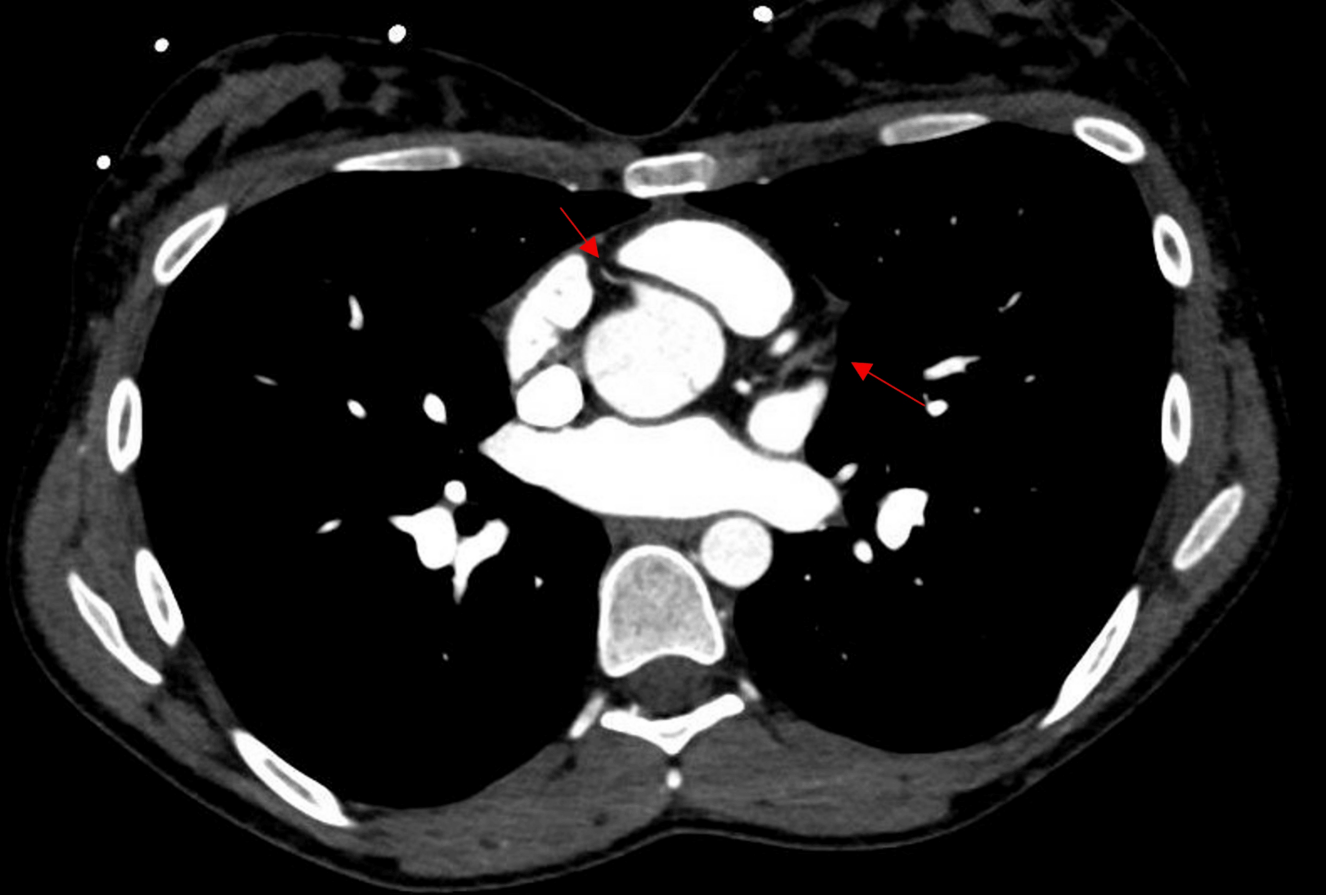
CT angiogram of the coronaries showing patent coronary arteries
